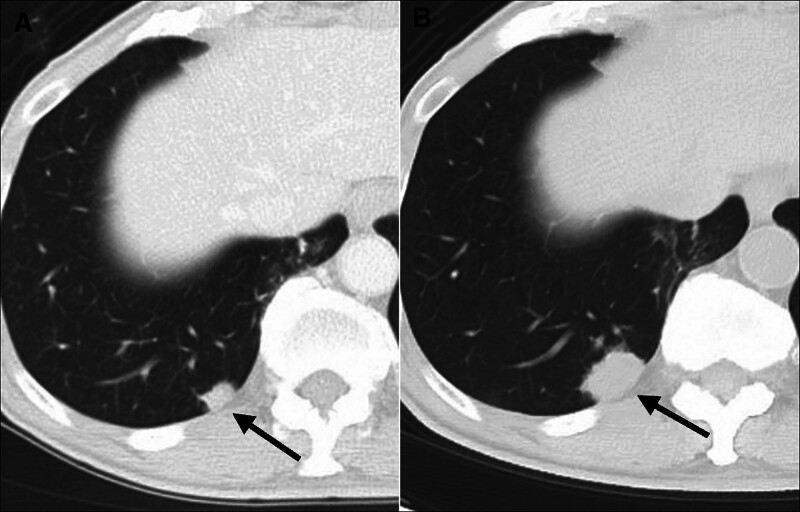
Radiological progression of pulmonary oligometastatic lesion following pancreatectomy. (A) Chest computed tomography (CT) at 25 months post-pancreatectomy revealed a 10-mm solitary solid nodule in the right lower lobe (black arrow), suggestive of pulmonary metastasis or primary lung cancer. (B) A follow-up CT 2 months later showed progressive lesion growth to 20 mm in diameter, indicating the need for surgical intervention.
